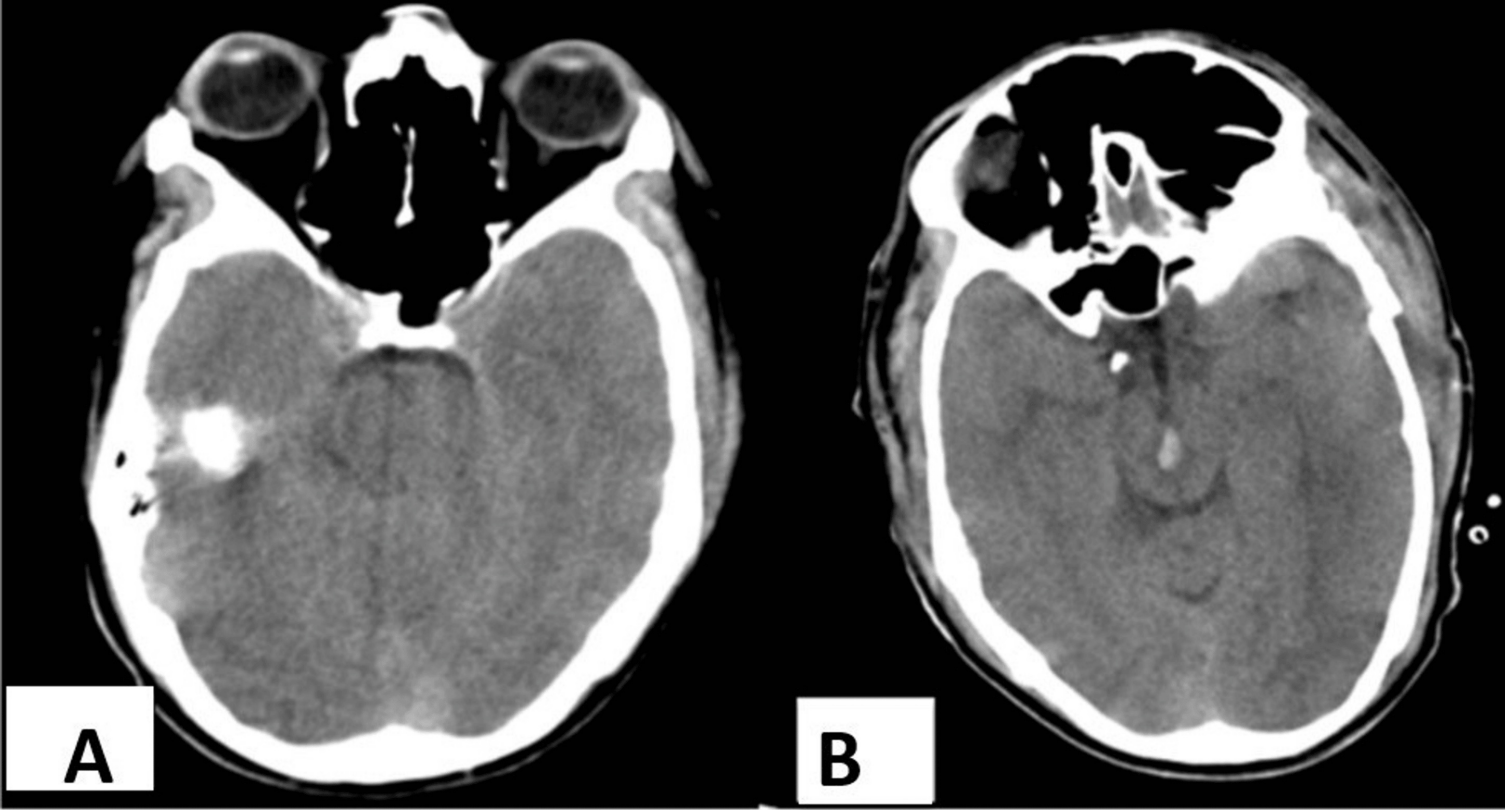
Comparative plain CT scan of brain. (A) Before surgery focusing on brainstem showing no signs of hemorrhage. (B) After surgical evacuation, due to possible descending transtentorial herniation, a newly developed Duret hemorrhage.
